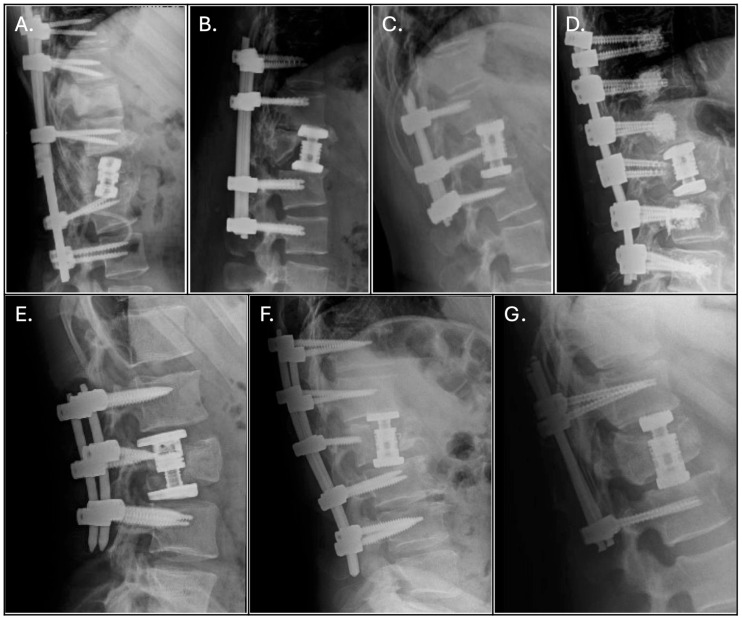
Postoperative XR Imaging. Each postoperative X-ray corresponds with the preoperative MR imaging in the same lettered panel in Figure 1. (A) Posterior fusion from T10 to L4 with L2 corpectomy and cage spanning L1-L3. T12 compression fracture persists with moderate height loss and retropulsion (3 months post-op). (B) Partial L1 corpectomy with anterior strut graft and posterior fusion from T11 to L3. Residual L1 fracture fragment remains. Other vertebral bodies are aligned with preserved height and disk spaces (post-op day 4). (C) L1 corpectomy with posterior fusion from T12 to L2. Vertebral alignment and heights are stable compared to prior imaging (3 months post-op). (D) Partial L3 corpectomy and posterior fusion from T12 to L5. Cement augmentation is present at screw tips. L3 compression fracture and L1 endplate deformity are stable. Disk space loss persists at L5-S1 (3 months post-op). (E) Partial L3 corpectomy and posterior fusion from L2 to L4. L3 burst fracture remains moderately compressed with mild retropulsion and anterior cortical displacement (2 months post-op). (F) Posterior fusion from T11 to L3 with L1 partial corpectomy and cage spanning from endplates of T12 to L2 (6 weeks post-op). (G) Posterior fusion from T12 to L2 with L1 corpectomy.
